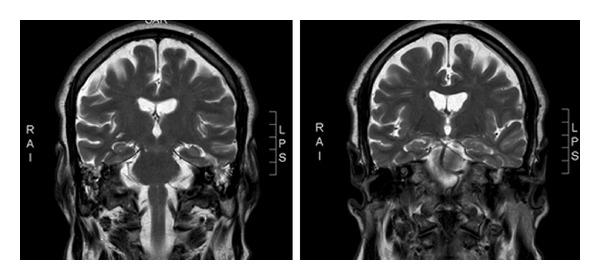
MRI showing areas highly suspicious of herniation. This was confirmed intraoperatively.
